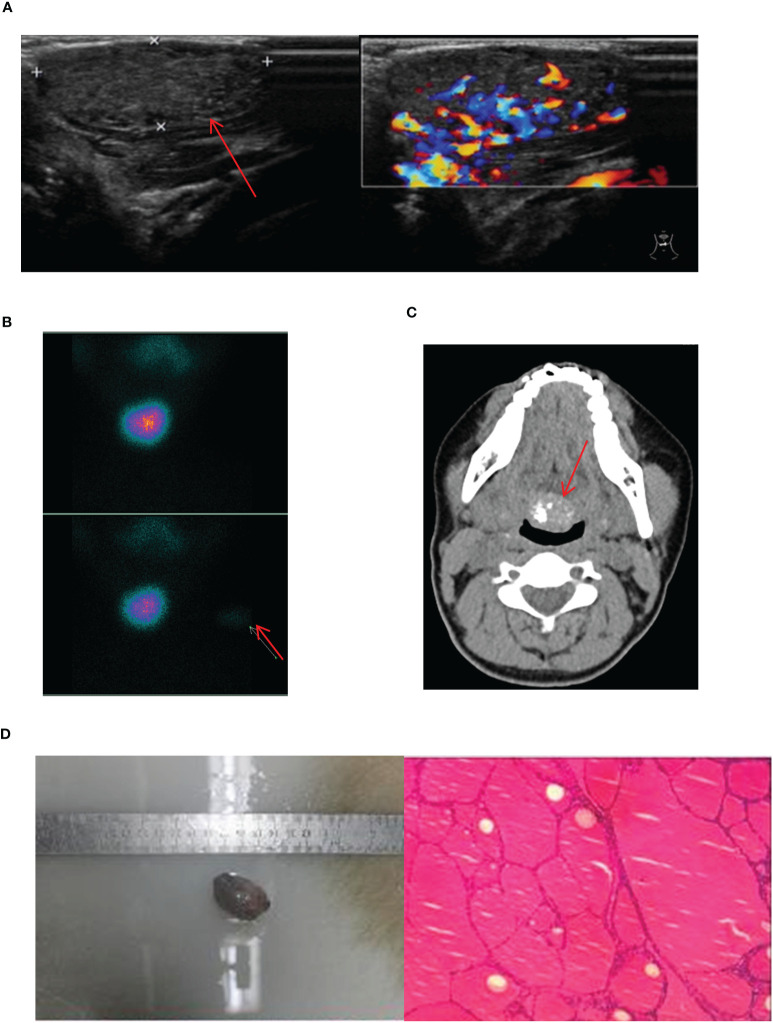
Imaging manifestations and pathological findings of submandibular ETG. (A): The thyroid ultrasound showed a hypoechoic glandular echo with clear boundaries, regular shape. The Color Doppler flow imaging showed abundant blood flow signals in the ETG. (B): Static thyroid imaging showed abnormal circular radioactive distribution under the mandible. (C): CT showed a soft tissue density shadow of nodules with clear boundaries in front of the trachea under the mandible, which was obviously enhanced after enhancement. (D): Postoperative pathology indicated the thyroid tissue. (C, D) were of the same patient.
